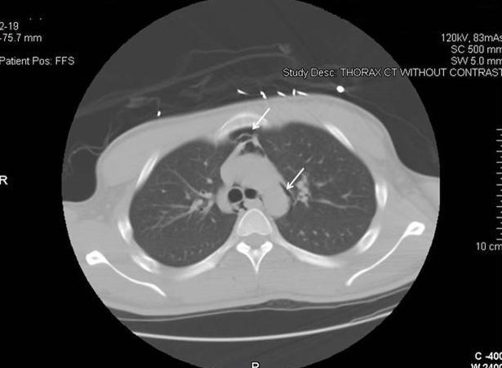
Computed tomography of the chest showing air in the mediastinum, ie pneumomediastinum.
